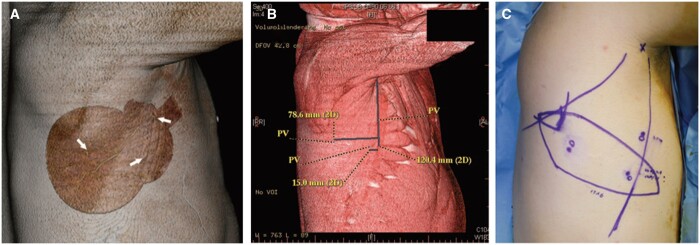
Perforator localization technique as shown by Mun et al. (A) Three localized perforators (arrows); (B) Perforators visualized in the volume-rendered image; (C) Mapped perforators were translocated to the patient and the flap was designed and positioned appropriately. Dots represent CT-marked perforators and open circles represent the Doppler-based perforator locations (used with permission of Wolters Kluwer Health, Inc.).^17^
